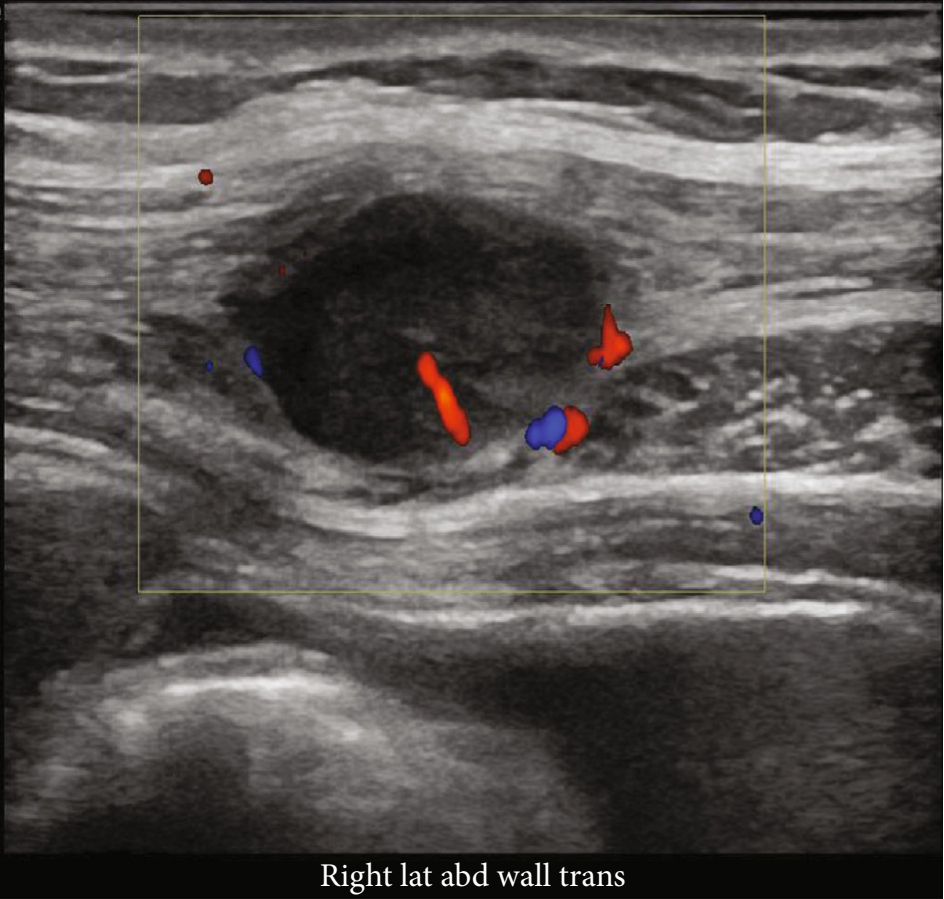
Doppler ultrasound confirming the subcutaneous nodule with internal vascularity and distortion of associated subcutaneous tissue planes, performed concurrent with image-guided biopsy.
